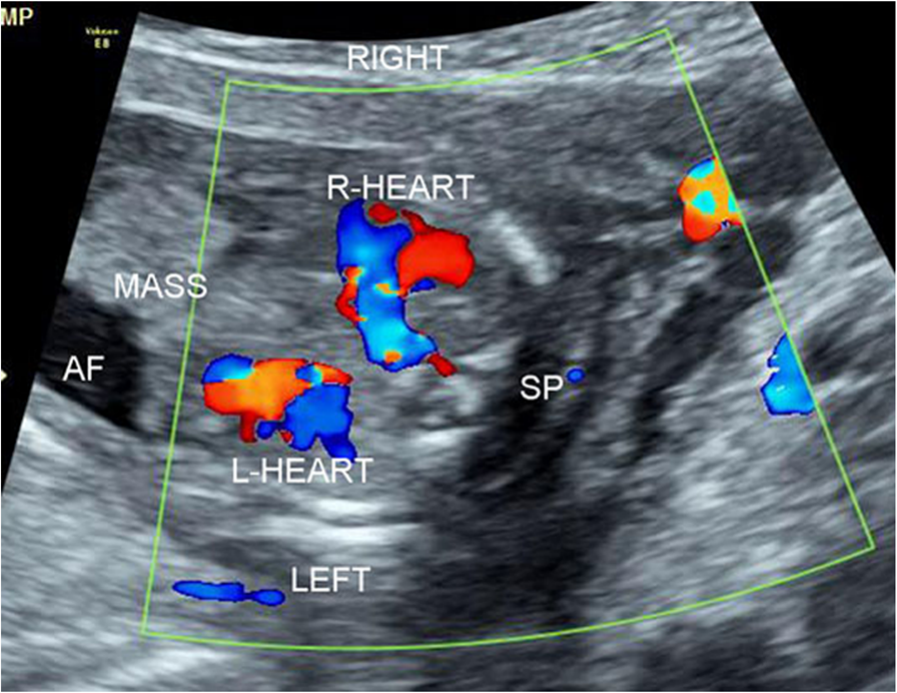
Color Doppler demonstrated pulsive color signals in the heart-like structure at the lowest end of the mass within the fetal left chest. The heart of the fetus was demonstrated on the right chest. AF, amniotic fluid; Sp, spine
